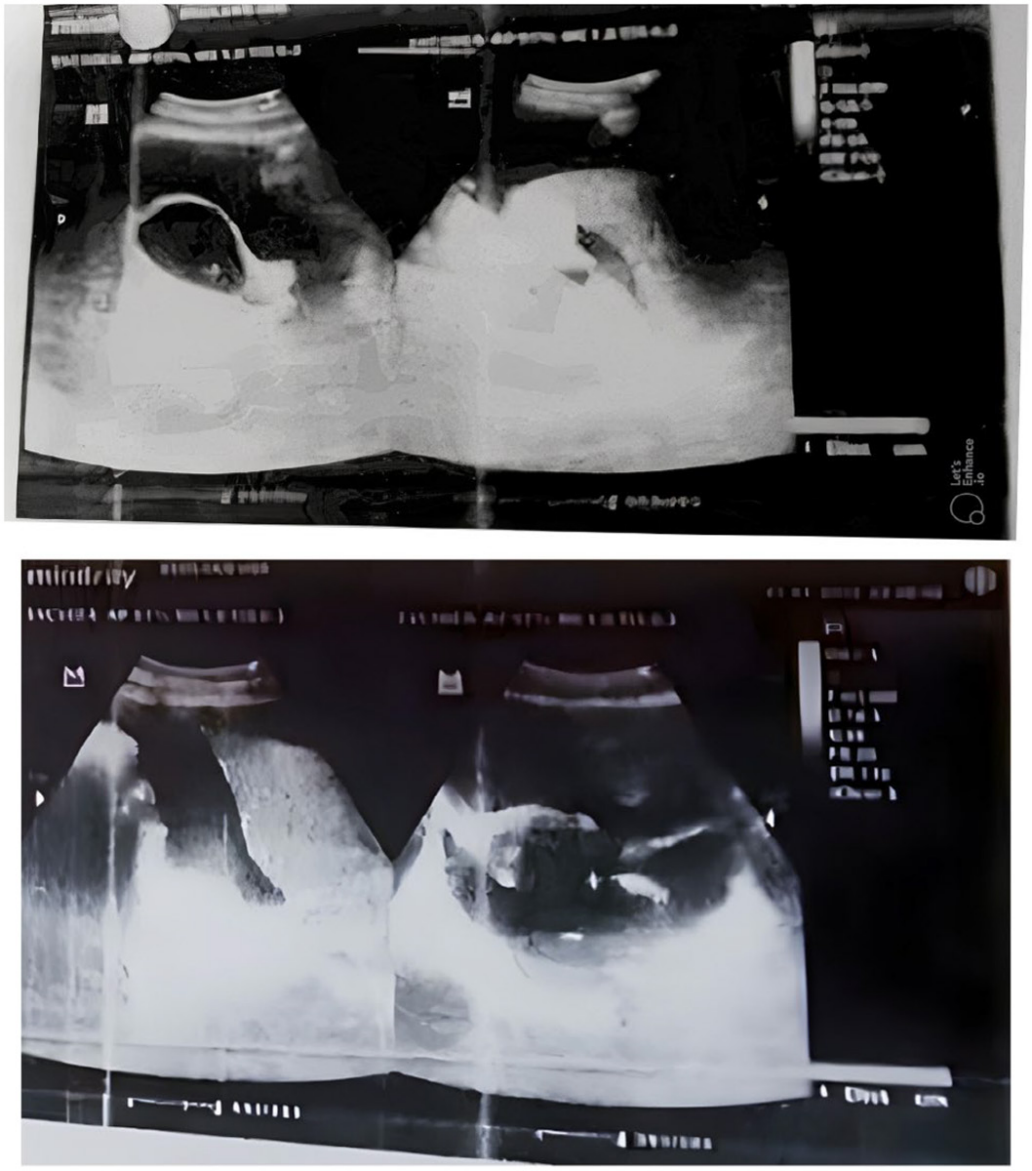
An abdominal echocardiogram showing free turbid fluid in the abdominal cavity.
